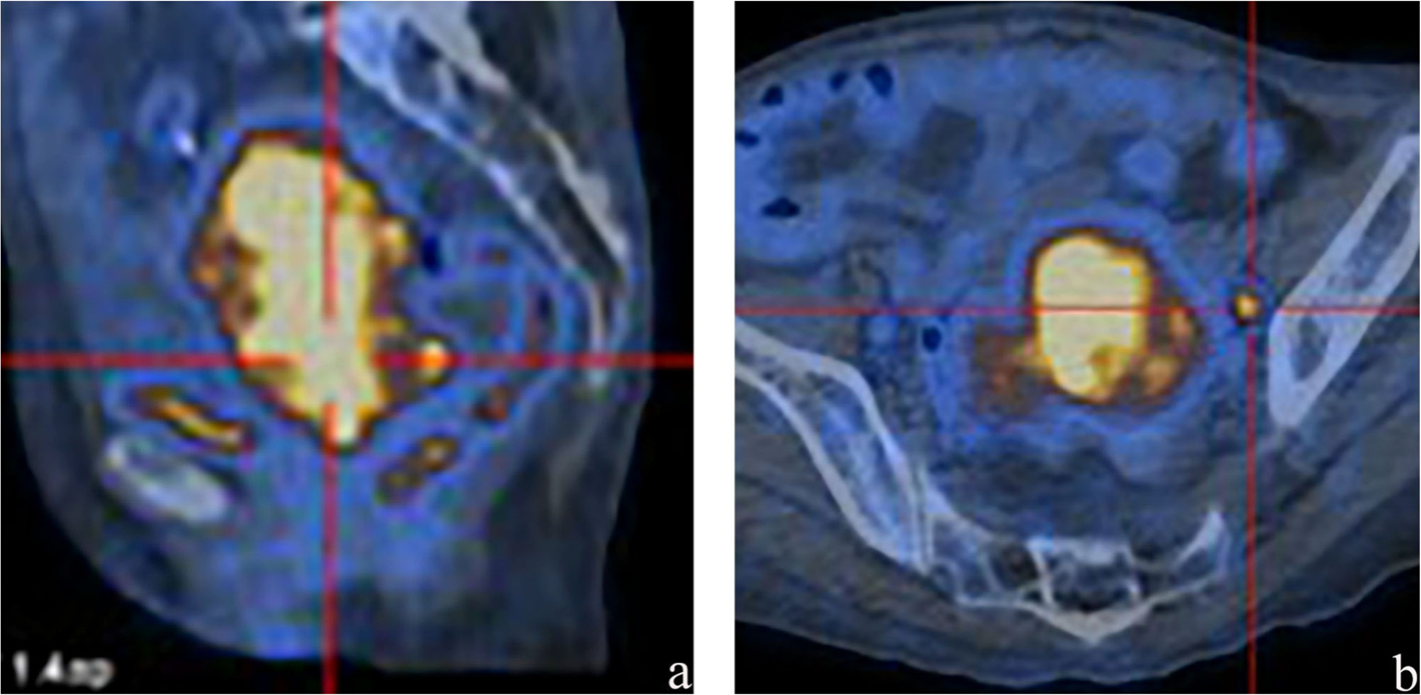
PET/CT images. a Sagittal PET/CT fusion image shows abnormal FDG accumulation in the uterus, and the SUVmax is 24.8. b Axial PET/CT fusion image shows abnormal FDG accumulation in the pelvic lymph nodes, and the SUVmax is 19.3
